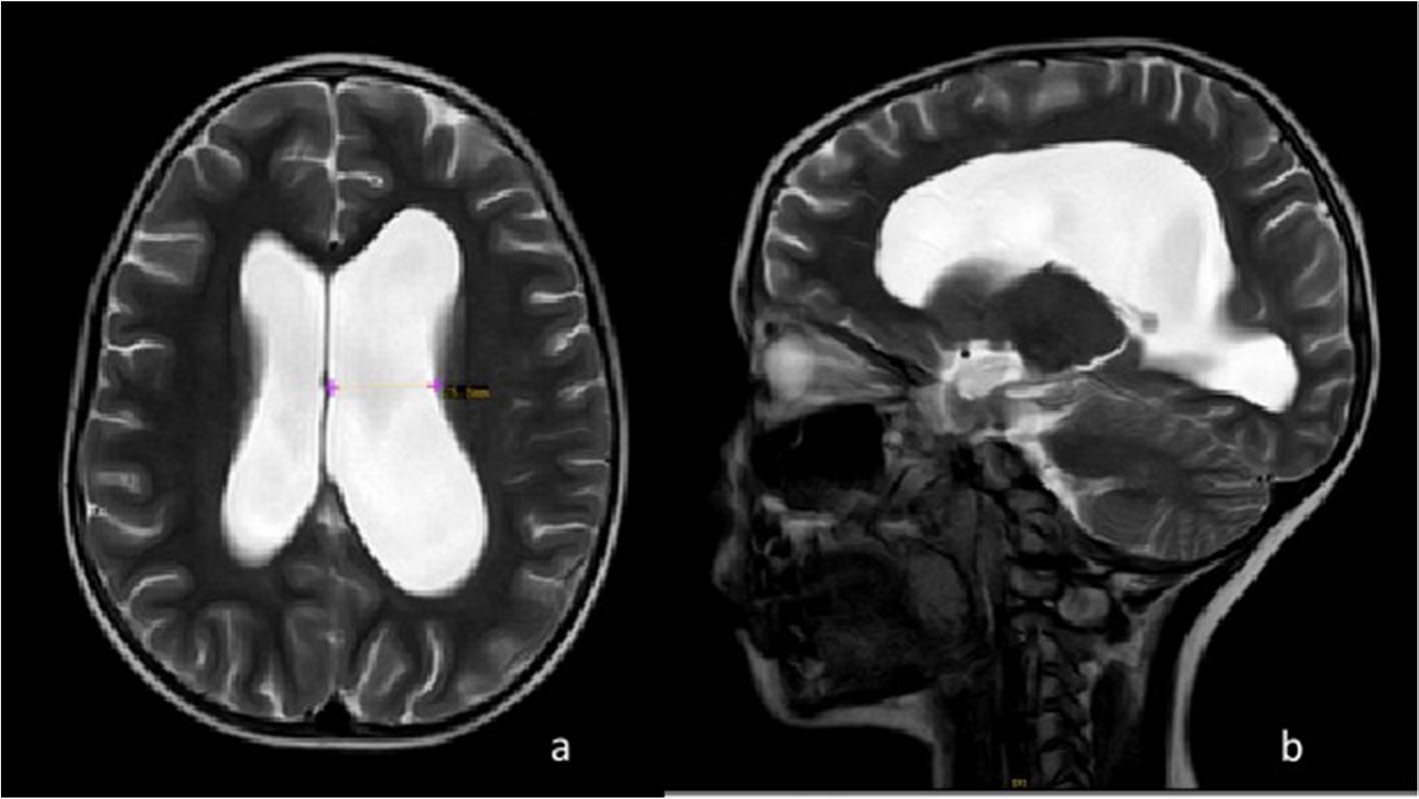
Brain MRI (T2WI) showed dilated ventricles, especially the left lateral ventricle
